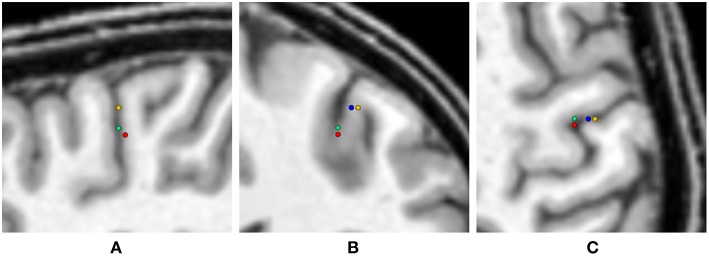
Visualization of the source locations with maximal GoF for the uni- and multivariate distributions overlaid on the T1-MRI (blue - skin, green - skull, yellow - CSF, gray matter, white matter, red - multivariate). From left to right showing sagittal (A), coronal (B), and axial slice (C). In the sagittal slice (A) the visualizations for skin and CSF, gray matter, and white matter are overlaying.
